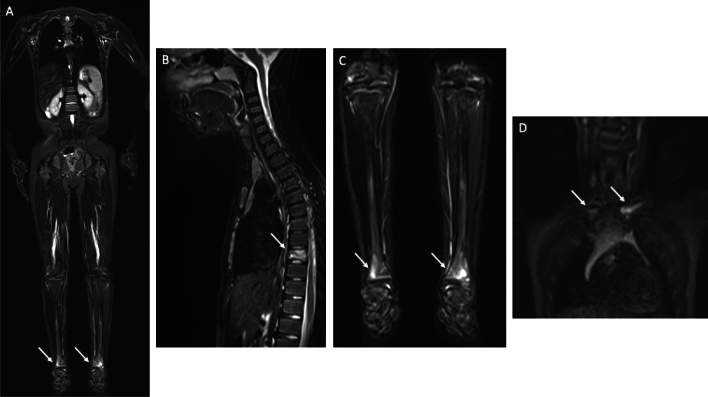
WB-MRI in a 9-year-old boy with CRMO. WB STIR (a) and Cor STIR (c) plane images show bilateral BME of the meta-epiphyseal distal tibia. Sagittal STIR (b) plane image show a fracture of the D9 vertebral body. Cor STIR (d) plane show BME of the sternal end of bilateral clavicle, mostly on the left side
